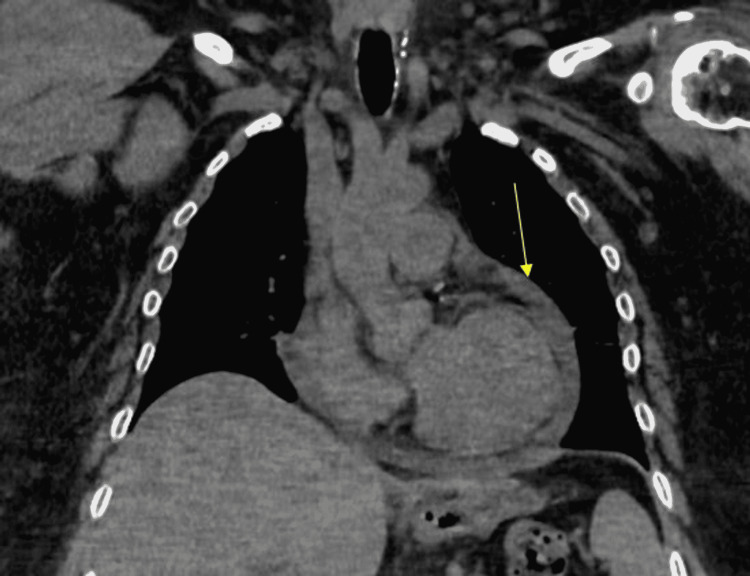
CT chest coronal reformatted image without contrast demonstrating small-to-moderate-sized pericardial effusion. Arrow pointing to pericardial effusion. CT: computerized tomography
